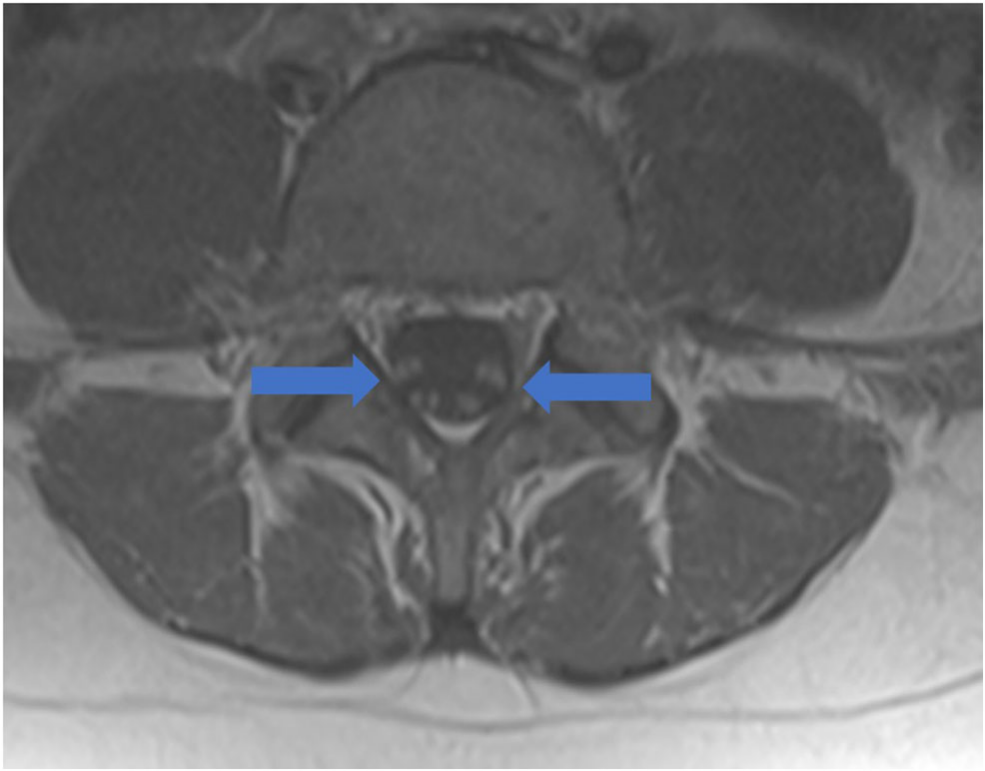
Guillain-Barré syndrome: Axial post-contrast T1 MRI of lumbar spine demonstrates diffuse enhancement without enlargement of the ventral and dorsal nerve roots (blue arrows).
